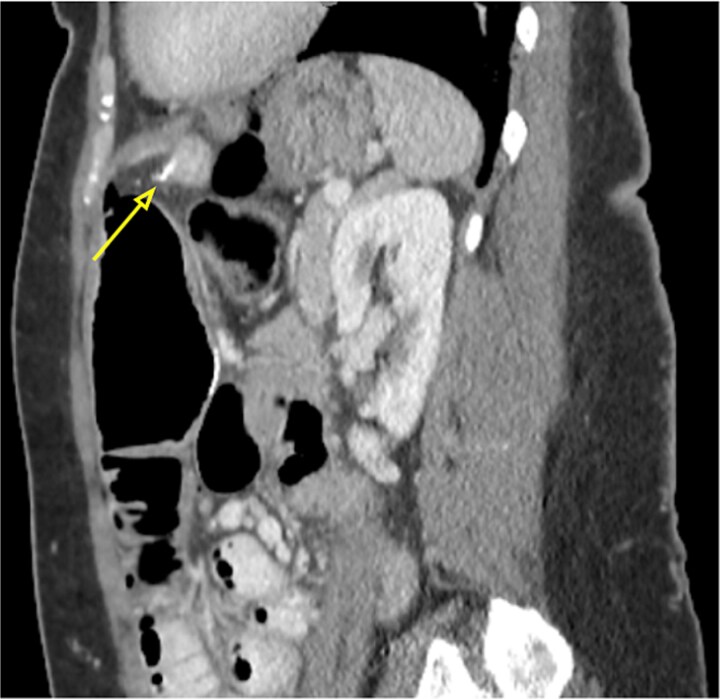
Sagittal abdominal CT scan showing the 4 cm hake fishbone (arrow).
